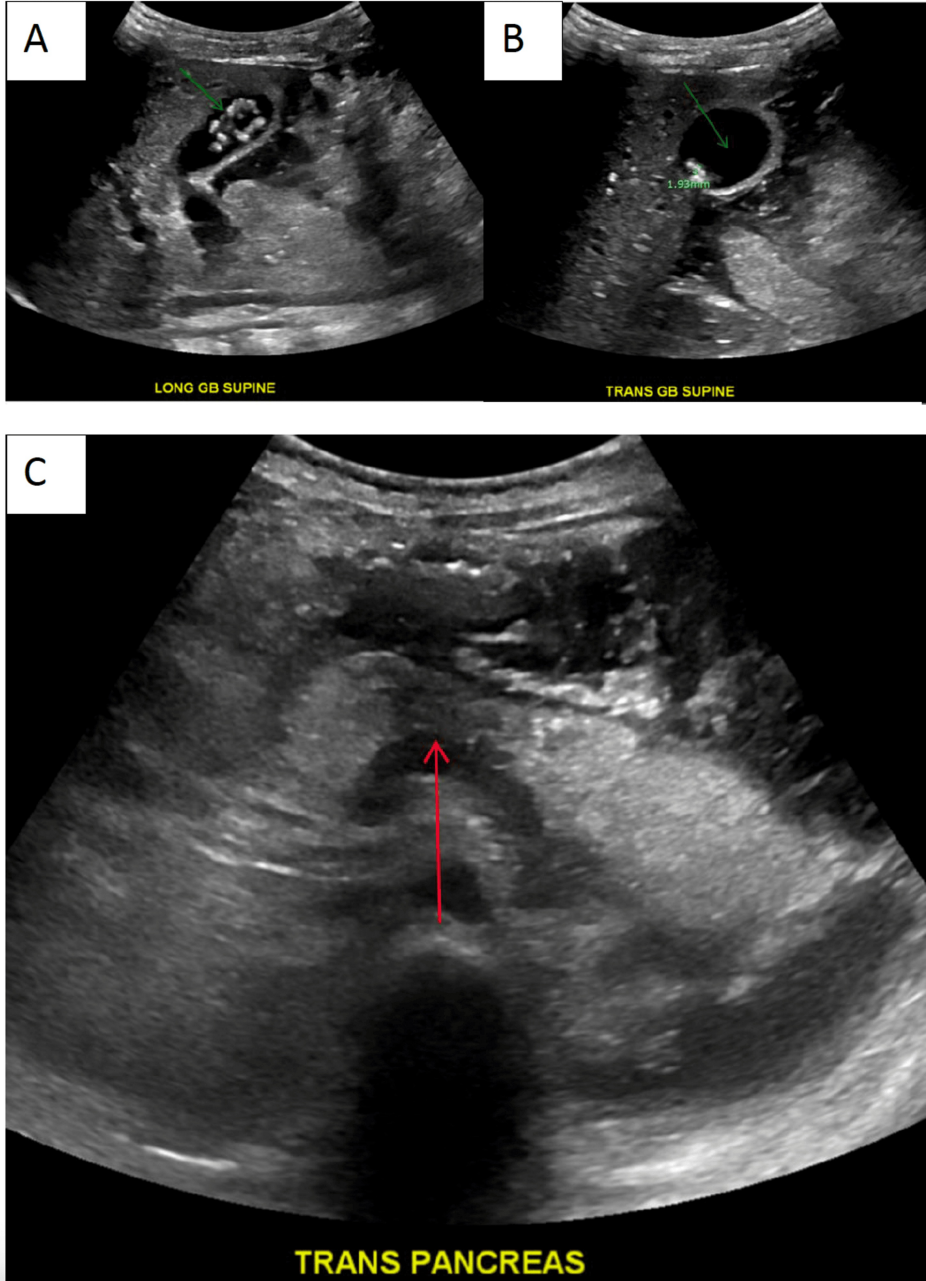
Ultrasound of the gallbladder and pancreas A) Long-axis view of the gallbladder revealing multiple gallstones (green arrow). B) Transverse view of the gallbladder (green arrow) revealing 2 mm stones (green measuring marker). C) Transverse view of the pancreas revealing cystic changes in the pancreatic body (red arrow) concerning for necrotizing pancreatitis.
